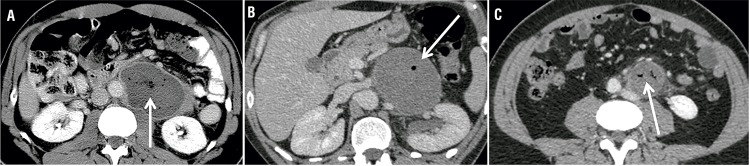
Abdominal contrast-enhanced CT-scan axial cut showing gas-containing retroperitoneal mass (white arrows).
